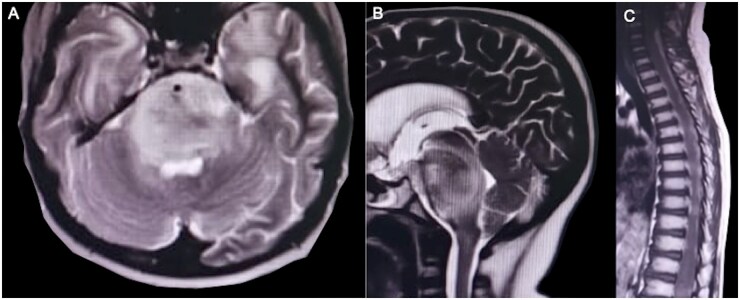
(A-C) Magnetic Resonance Imaging of Case 1 (14-year-old patient, 8 years after adjuvant RT for CP). The quality of the selected images is related to the copy of the images from film prints. Axial T2-weighted image shows an expansive mass infiltrating the entire cross-section of the basis pontis, engulfing the basilary artery in the prepontine cisterna, and extending to the middle cerebellar peduncles, fulfilling the imaging criteria of DIPG (A). Sagittal T2-weighted shows the cranial extension of the mass to the midbrain with mass effect of the cerebral aqueduct and secondary obstructive hydrocephalus with visible dilatation of the third ventricle (B). Follow-up MRI performed in the setting of acute paraplegia, i.e., 5 months after completion of re-irradiation, demonstrating leptomeningeal dissemination on sagittal contrast-enhanced T1-weighted images of the spine (C).
